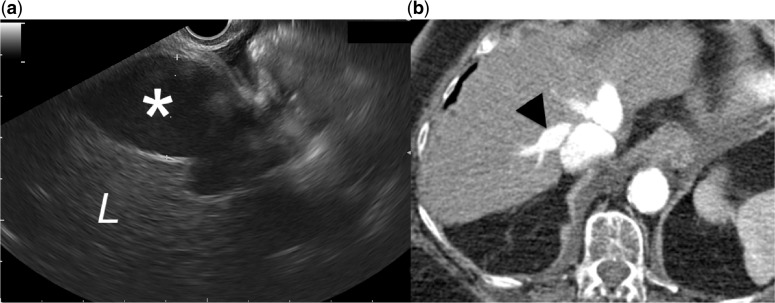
(a) Endoscopic ultrasound signs of right-sided heart failure: dilation of the hepatic veins and inferior vena cava (asterisk) to 25 mm. The endoscope is positioned 1–3 cm beyond the gastro-oesophageal junction and rotated clockwise. (b) Upper abdominal contrast-enhanced computer tomography section reveals retrograde opacification of the dilated hepatic veins (arrowhead), which is a specific sign of right-sided heart failure.
